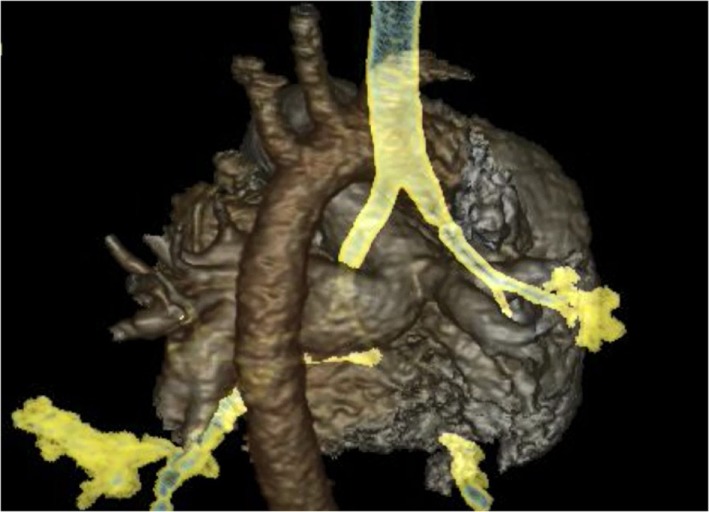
CT angiogram 3D reconstruction of left pulmonary artery sling.
